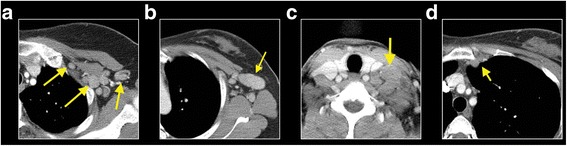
The representative CT images of the regional lymph node enlargements.*Yellow arrows* indicate the presence of the enlarged lymph nodes in axillary level I-III (a), a lymph node larger than 2cm in level I (b), enlarged supraclavicular lymph node (c), and an internal mammary node (d)
